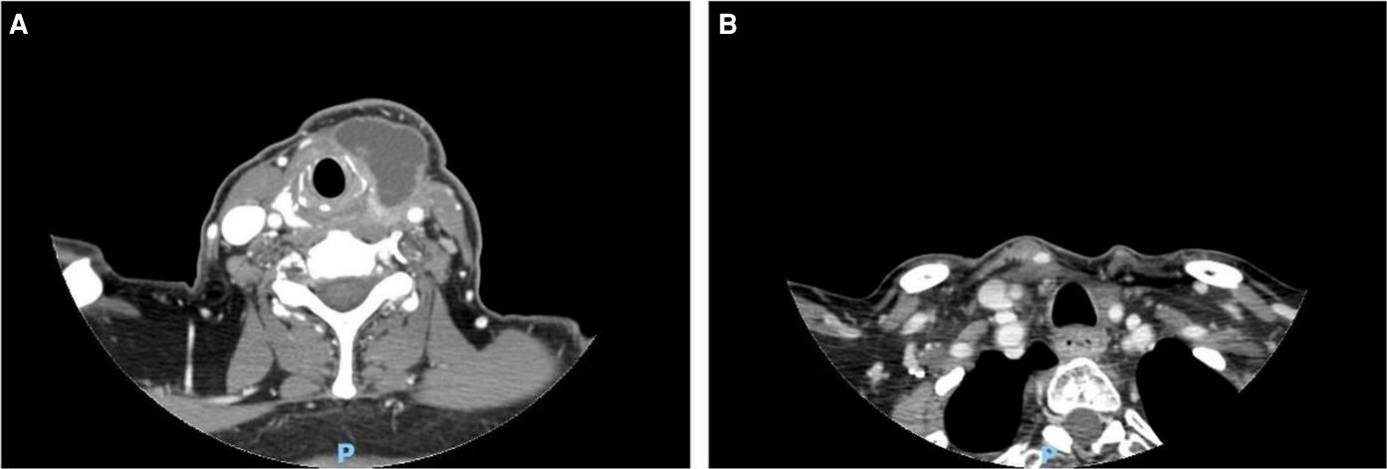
(A) Axial CT scan demonstrating a locoregional relapse of ATC: a large cystic formation at paratracheal site, displacing the left submandibular gland and infiltrating trachea, bordering muscles and skin. (B) Axial CT scan of a locoregional relapse of ATC treated with chemo-immunotherapy combined with radiotherapy, demonstrating a nearly complete radiological response. Abbreviations: ATC, anaplastic thyroid cancer; CT, computed tomography.
